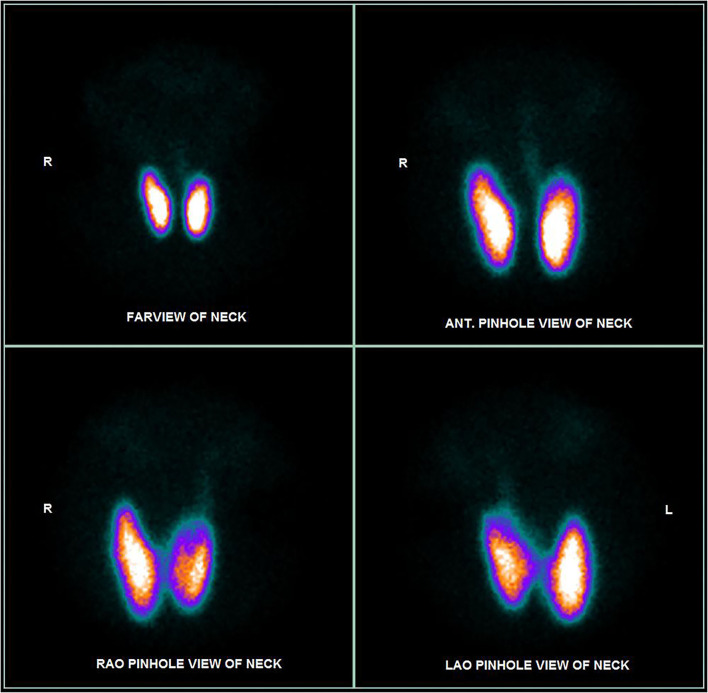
Thyroid ultrasonography at the diagnosis of Graves' disease of our patient (left panel: both lobes without Doppler; mid panel: right lobe with Doppler; right panel: left lobe with Doppler).
